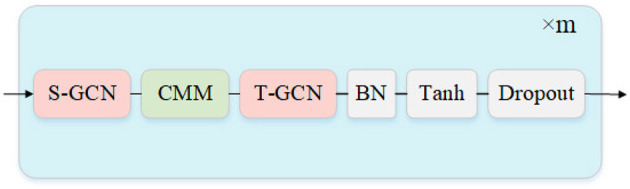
The structure of fine-tuning model. CMM denotes causal mask matrix that improve the T-GCN. The hyperparameter m denotes the cascade number of “CT-GCL”.
